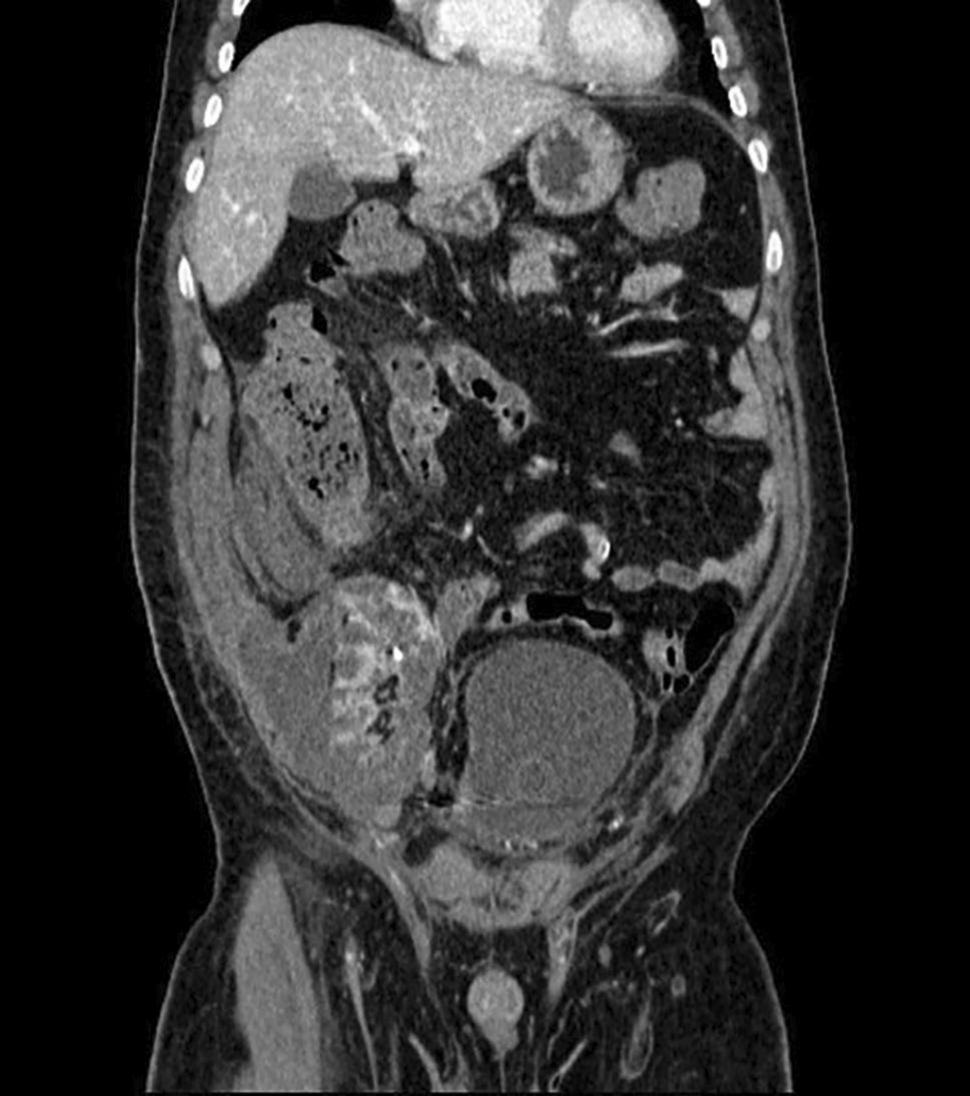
CT angiography confirms renal ischemia of lower pole of transplanted kidney
